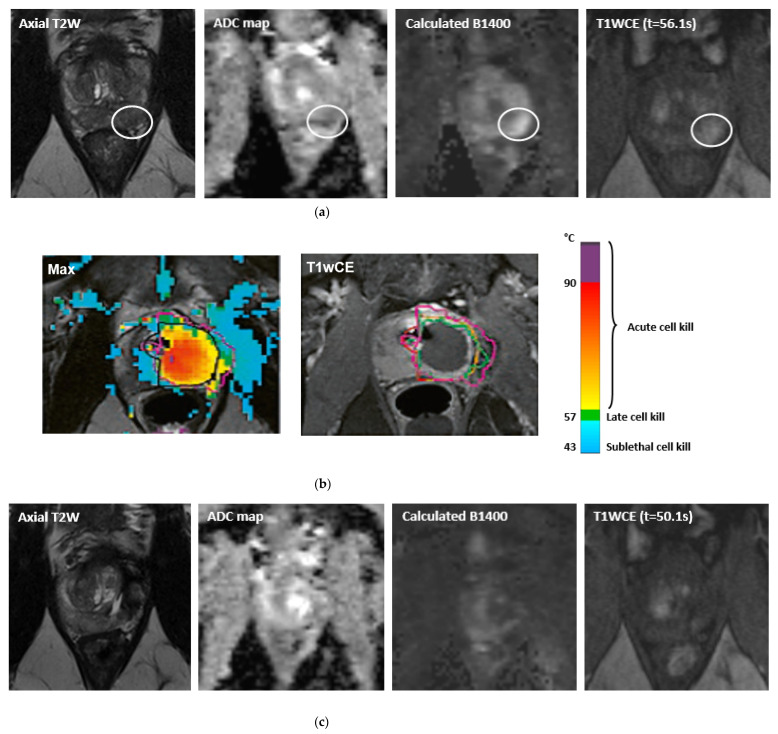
A 60-year-old patient with a PSA level of 15 ng/mL and a Gleason 3 + 4 = 7 prostate cancer in the left peripheral zone (PI-RADS 4). The maximum lesion dimensions in AP, LR and CC are 43, 46 and 35 mm. (a) Pre-treatment multiparametric MR images: Axial T2W image, high *b* value and contrast-enhanced image, respectively. (b) MR images demonstrating hemiablation on the left with transurethral ultrasound ablation (TULSA). (c) Post-treatment multiparametric MR images at two year follow-up (PSA level of 3.1 ng/mL): Axial T2W image, ADC map, high *b* value and contrast-enhanced image, respectively. There is no evidence of residual disease or recurrence.
